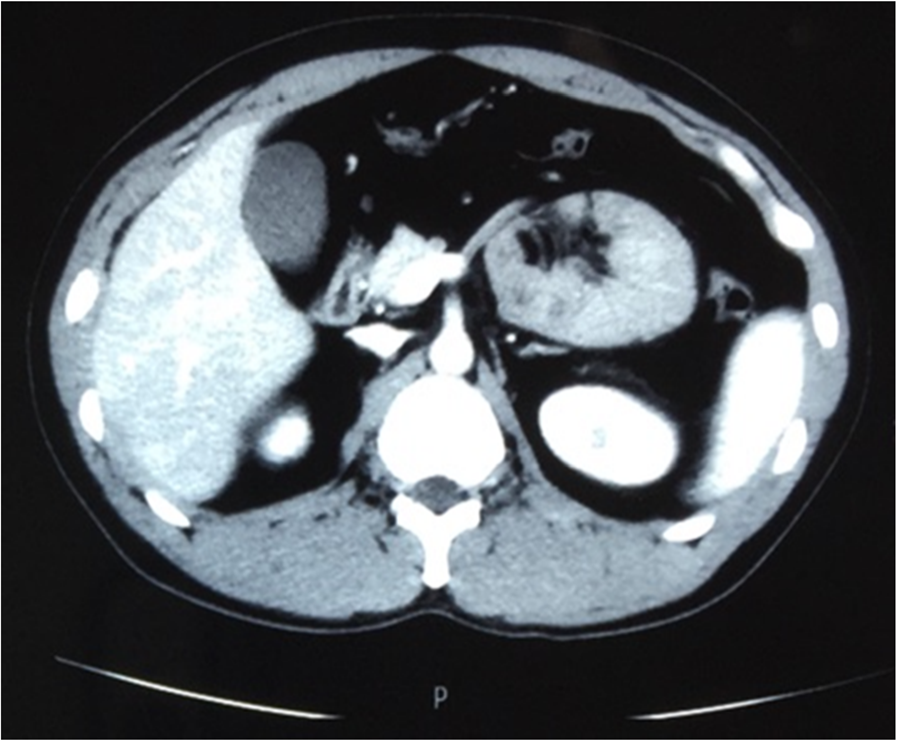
Transverse view of CT image showing the cluster of small intestine and its mesentery
